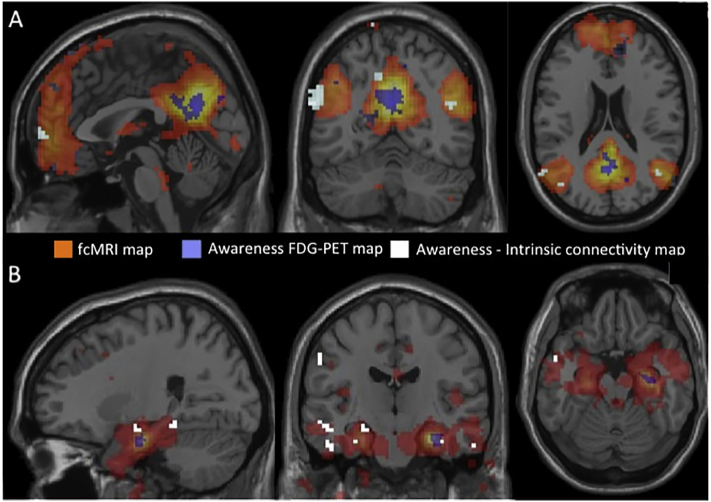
Overlay of the awareness-FDG-PET and awareness-intrinsic connectivity maps (color coded in white and purple) on top of the intrinsic connectivity map for the (A) PCC seed region respectively (B) MTL seed region elicited in the MCI patients (orange). (For interpretation of the references to color in this figure legend, the reader is referred to the web version of this article.)
